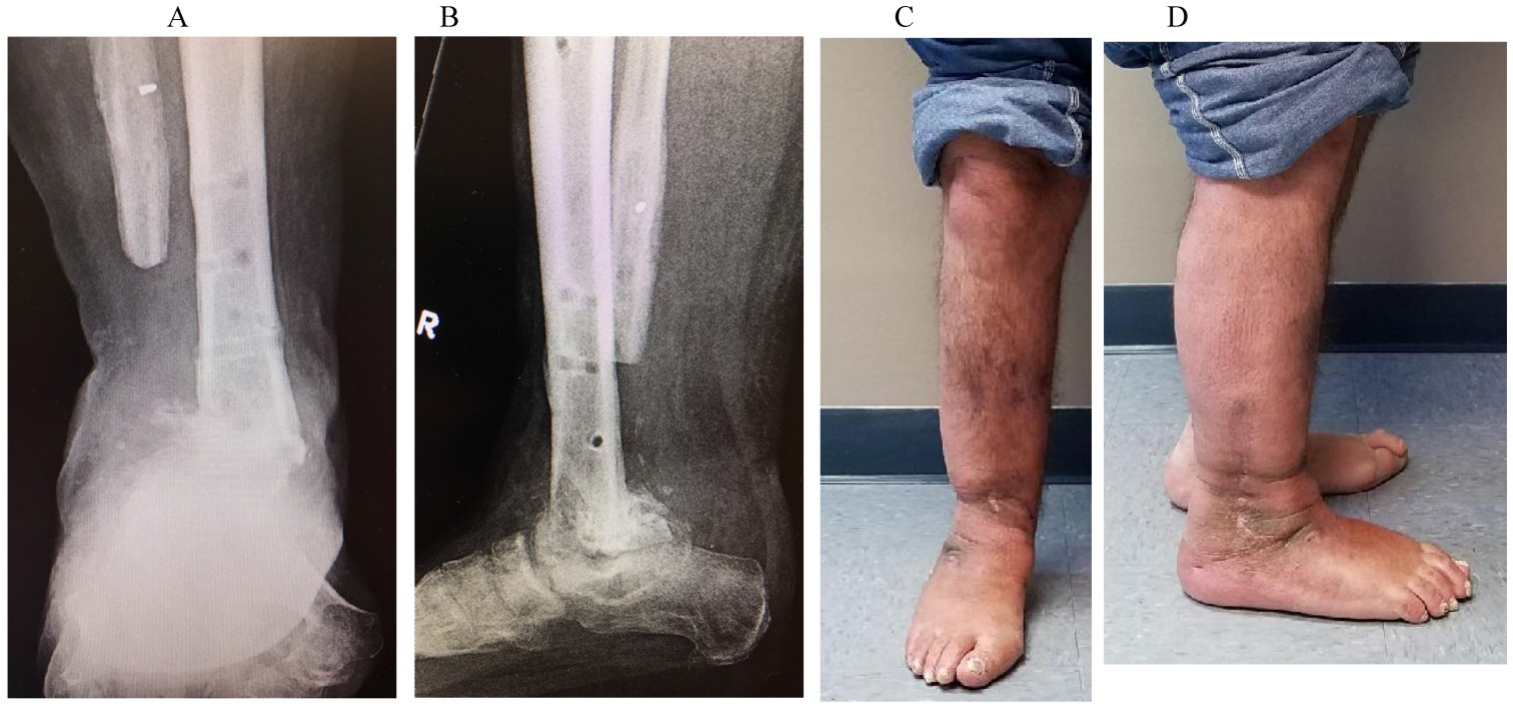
AP (A) and Lateral (B) radiographs with same day clinical appearance of extremity (C & D) 1-year following fusion demonstrating a solid tibiotalar fusion mass and well healed incisions with no evidence of infection recurrence.
